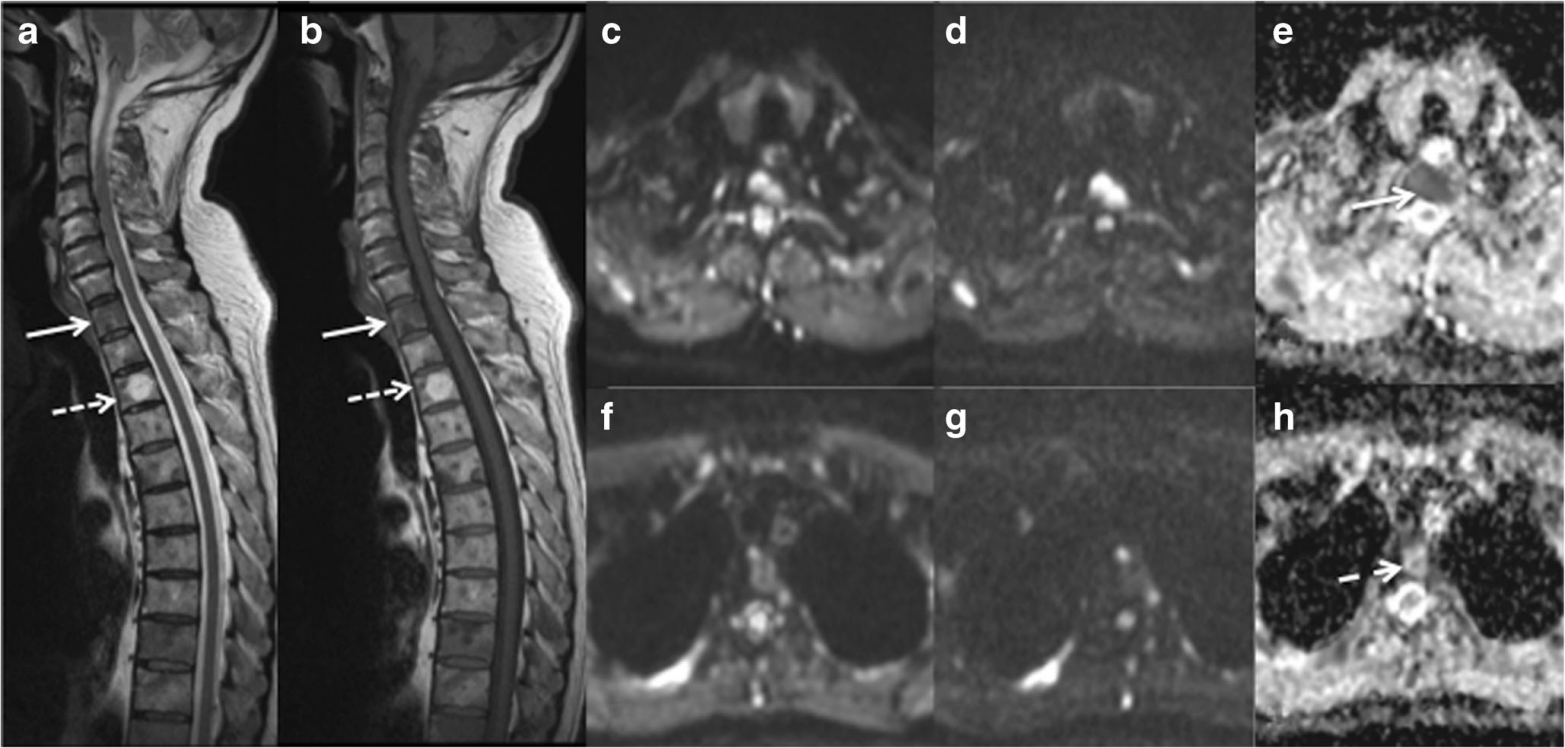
(a) Sagittal T_2_w and (b) T_1_w MRI of the cervico-thoracic spine shows a typical low signal metastasis (arrow) and a typical high signal haemangioma (dashed arrow). Corresponding axial diffusion-weighted MRI images of the metastasis (c b=50 s mm^-2^, d b=900 s mm^-2^, and e apparent diffusion coefficient (ADC) map) and of the haemangioma (f b=50 s mm^-2^, g b=900 s mm^-2^, and h ADC map) are shown. The metastasis exhibits restricted diffusion on the ADC map (e, arrow) but conversely the haemangioma has a high ADC (h, dashed arrow)
